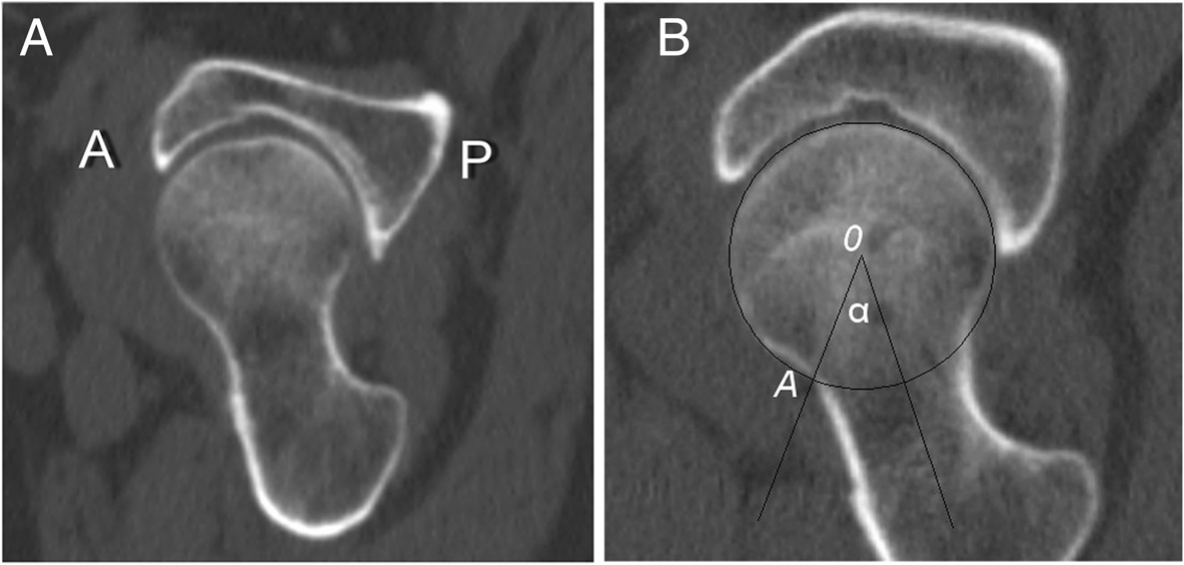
Reconstructed CT image of proximal femora and measurement of the alpha angle. (A) View of the oblique axial plane parallel to the axis of the femoral neck. (B) Measurement of the alpha angle; see the text for definitions of the various points.
